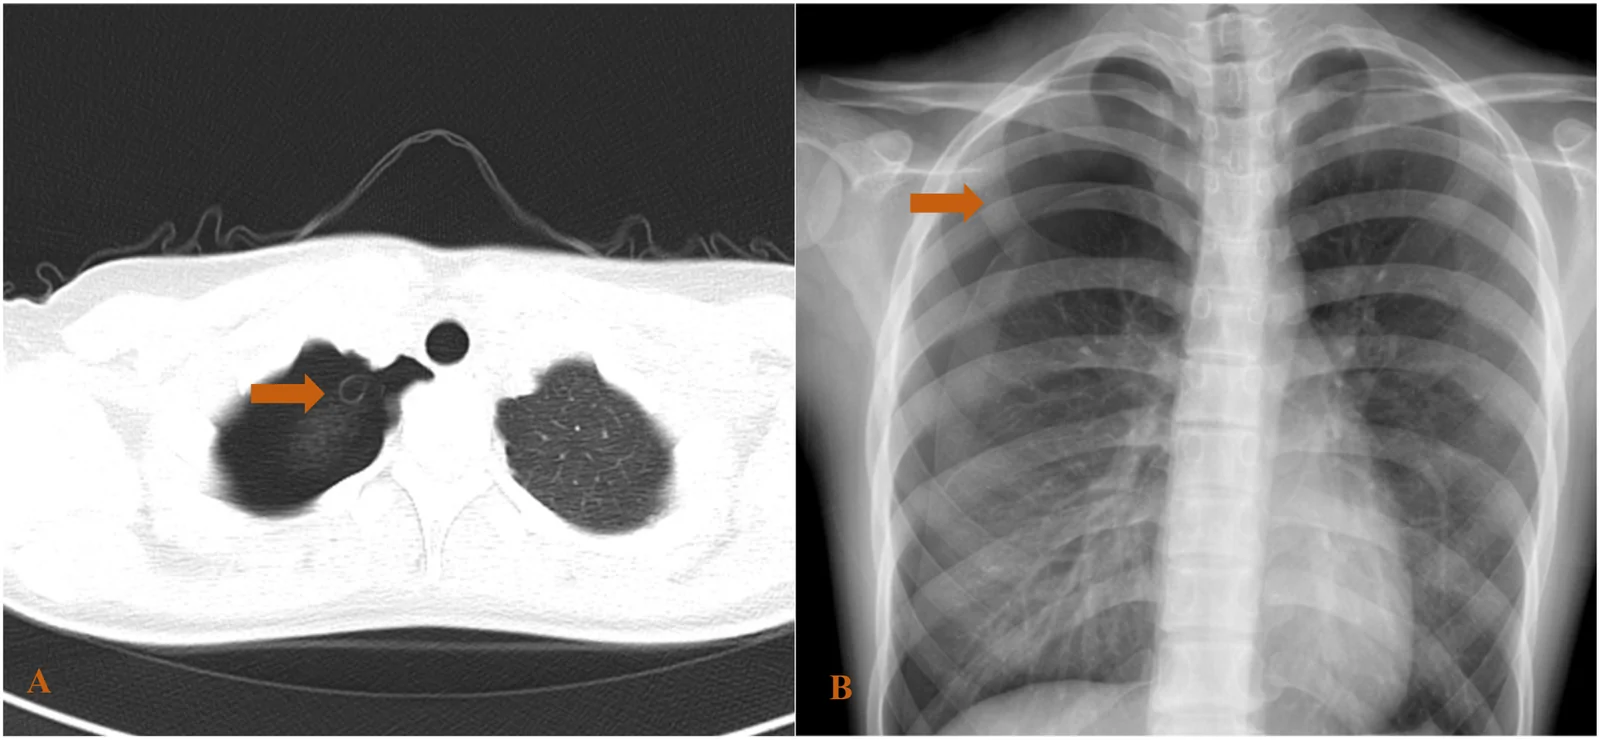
Preoperative chest imaging of the first surgery. (A) Chest CT showing right apical pulmonary bullae; (B) Chest x-ray demonstrating right spontaneous pneumothorax.
